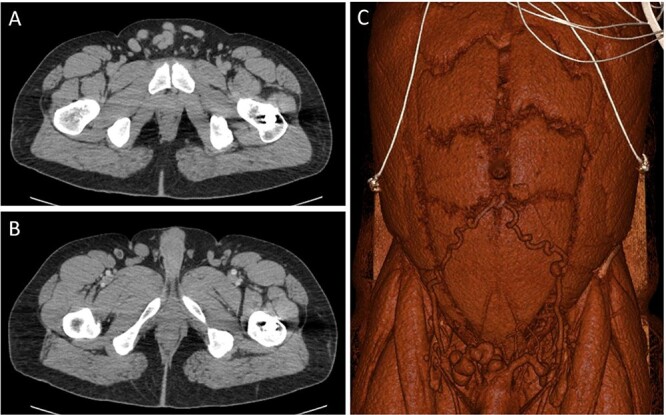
CT images through the pelvis show the dilated collateral veins (A) in the suprapubic region, initially thought to be a hernia. Solid white arrows (B) show the external pudendal vein confluences with the great saphenous veins. 3D reconstructed image (C) shows the dilated inferior epigastric and external pudendal veins, providing collateral flow around the stenosed left iliofemoral venous system.
